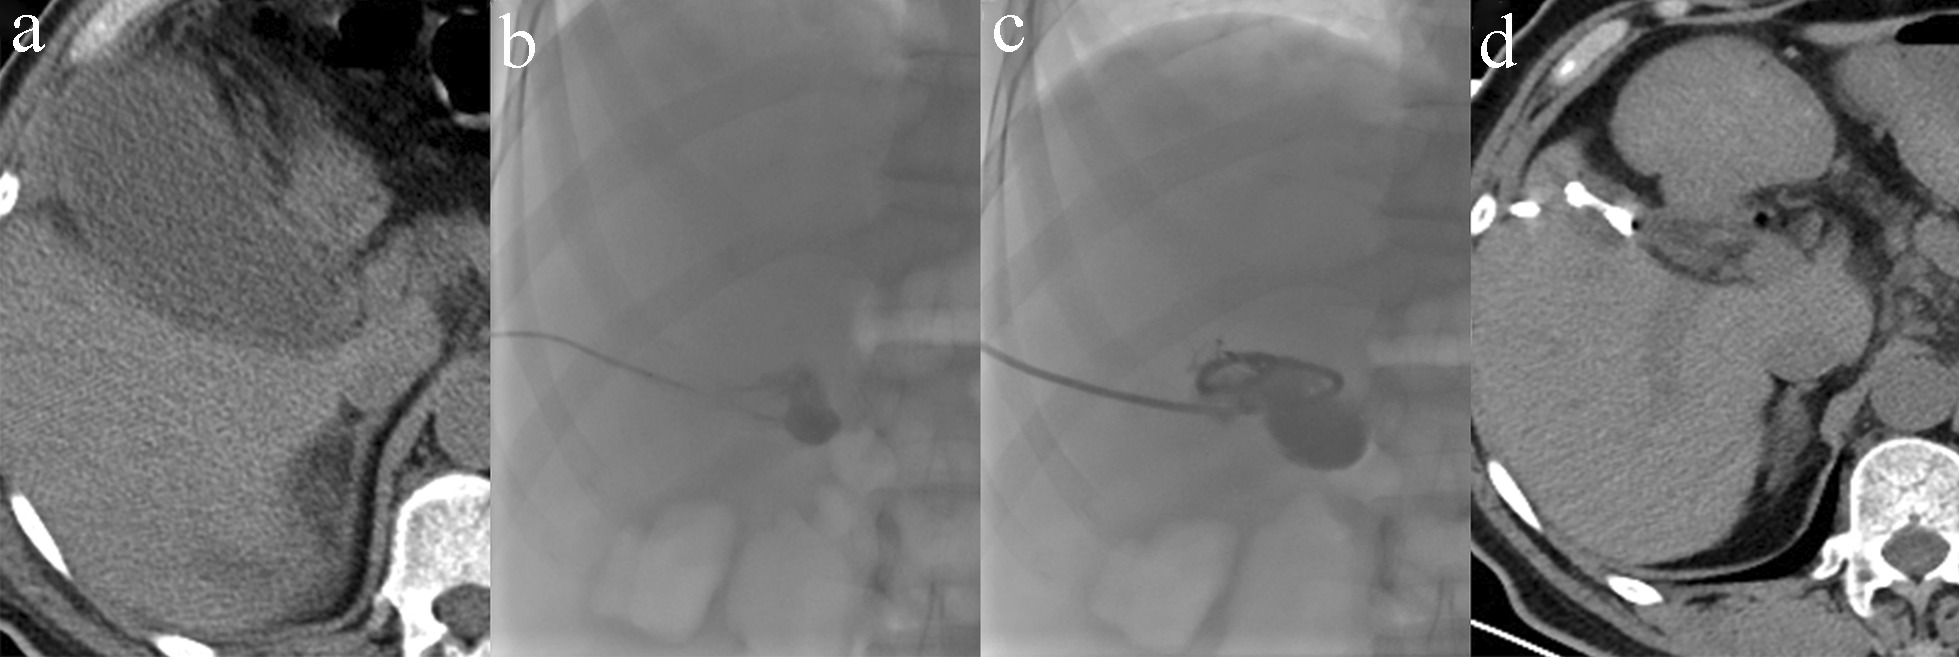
The procedure of percutaneous cholecystostomy. a A 59-year-old man was diagnosed with acute acalculous cholecystitis on the 7th day after colon cancer surgery. Computed tomography (CT) showed gallbladder dilatation and gallbladder wall thickening with peripheral exudation. b The patient could not tolerate cholecystectomy; therefore, percutaneous cholecystostomy was performed to relieve the patient's symptoms. Ultrasound guided percutaneous puncture of the gallbladder was successfully confirmed by contrast injection. c An 8F pigtail drainage catheter was introduced into the gallbladder under fluoroscopic guidance. d Three months later, CT showed that the gallbladder had retracted, and the surrounding exudation was absorbed. However, the patient was still unable to tolerate cholecystectomy and had a long-term indwelling PC tube
